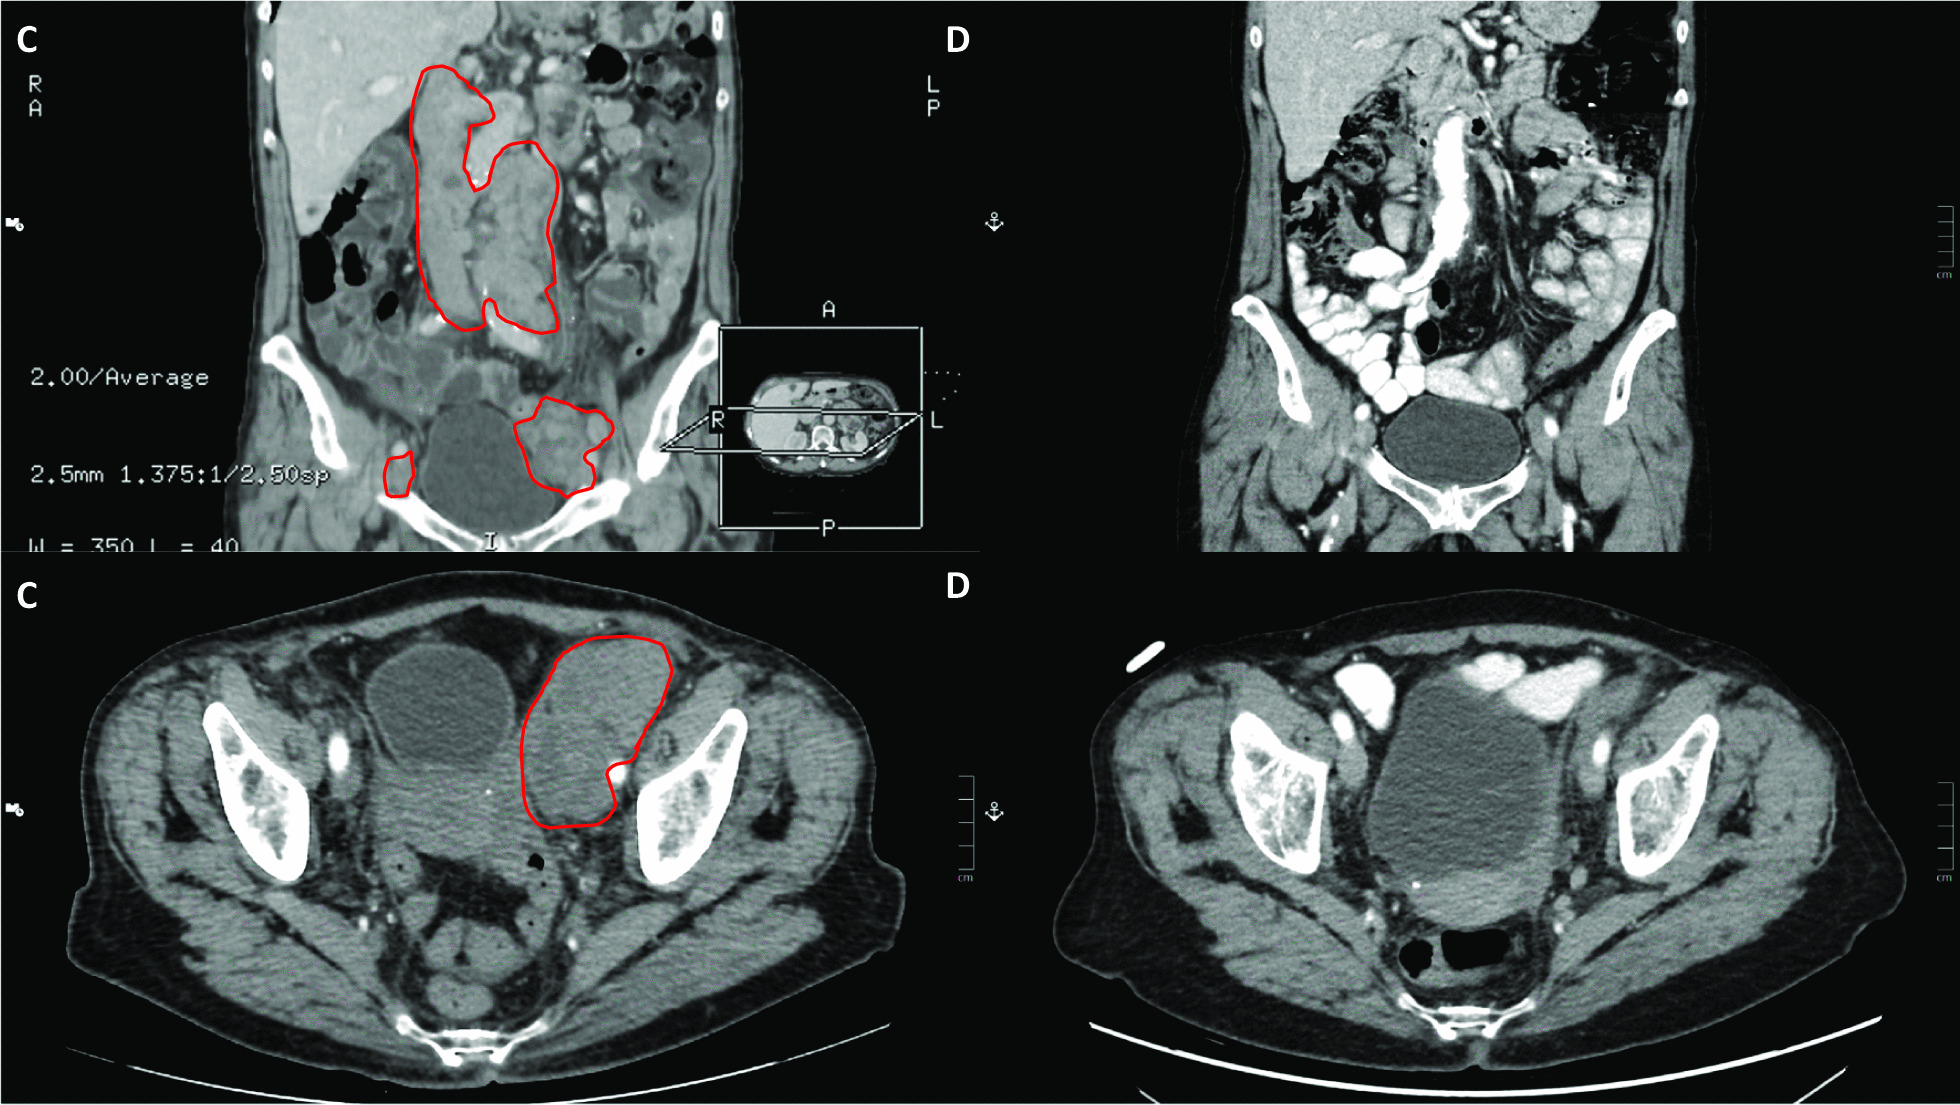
C CT scans of the abdomen and pelvis showing the lymph node conglomerates in the aortic and left parailiac regions, indicating the metastasis of the patient’s muscle-invasive urothelial carcinoma (September 2019). D CT scans of the abdomen and pelvis following atezolizumab therapy, showing the regression of the retroperitoneal and left parailiac lymph node conglomerates, indicating complete remission of the urothelial carcinoma metastasis (June 2020)
